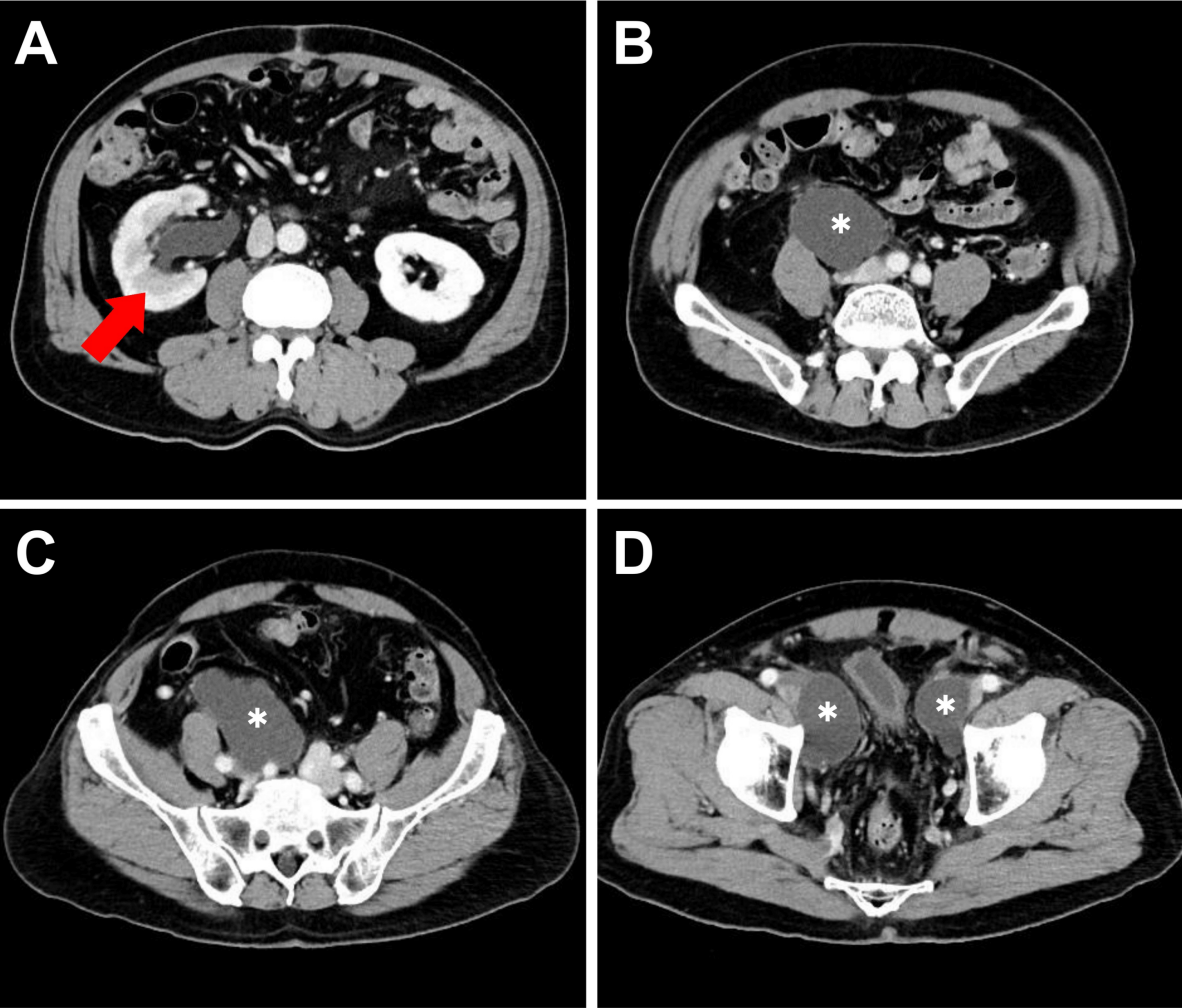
Contrast-enhanced abdominal CT in axial view one month after RaRP with PLND (A) Significant right hydronephrosis (red arrow). (B-D) Multiple lymphoceles (white asterisks) in the right common iliac region (compressing the right ureter), the left external iliac region, and the bilateral peri-vesical area. RaRP: robotic-assisted radical prostatectomy; PLND: pelvic lymph node dissection; CT: computed tomography
